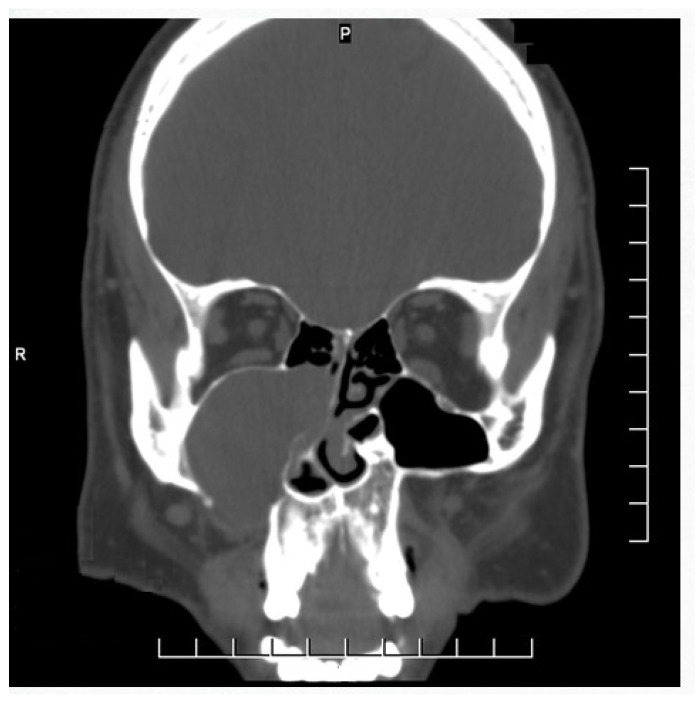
CT scan reveals a right opacified maxillary sinus with medial expansion leading to obstruction of the right nasal cavity and superior bulging causing encroachment on the floor of the orbit.
